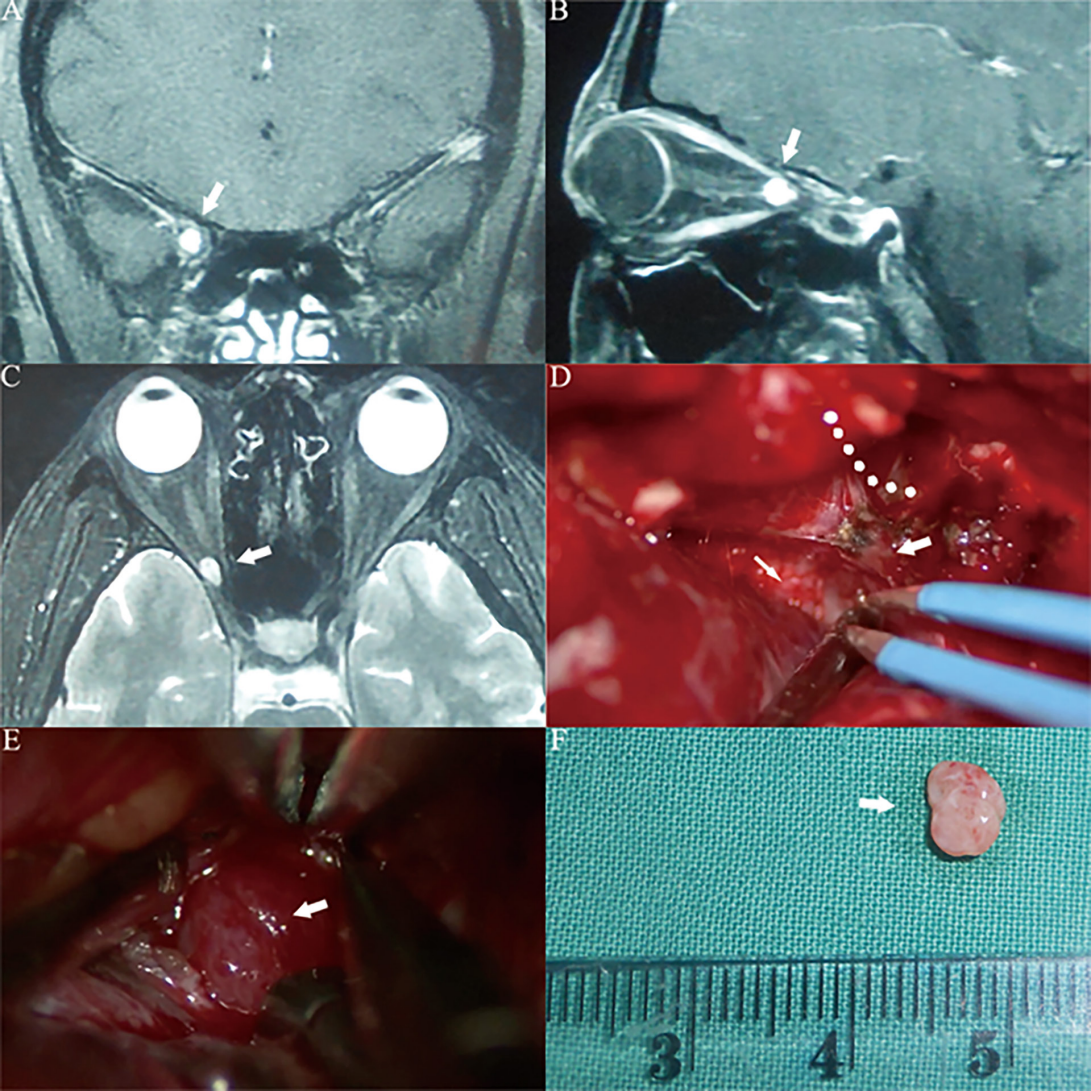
Case4. A 34-year-old female presented with her right eye vision loss for 2 months and sudden loss of right eye vision for 2 weeks. She was misdiagnosed as optic atrophy in the local hospital and was given hormone therapy. (A–C) MRI of the optic nerve showed a space-occupying lesion in the ring of the common tendon in the right orbital apex area, with uniform enhancement, and the tumor diameter was less than 1 cm (white thick arrow). (D) The tumor is located lateral the optic nerve. We used a right frontal-orbital approach craniotomy to remove the tumor. Abrasion of the optic canal bone shows the right optic nerve (white thin arrow), abrasion of the superior orbital fissure bone shows the right superior orbital fissure (white thick arrow), and the orbital periosteum at the orbital apex is incised lateral the superior orbital fissure along the dotted line. (E) It can be seen that the tumor capsule is intact, light red, and located lateral the right optic nerve (white thick arrow). (F) The tumor can be seen after complete resection. The actual tumor diameter is about 5mm (white thick arrow).
